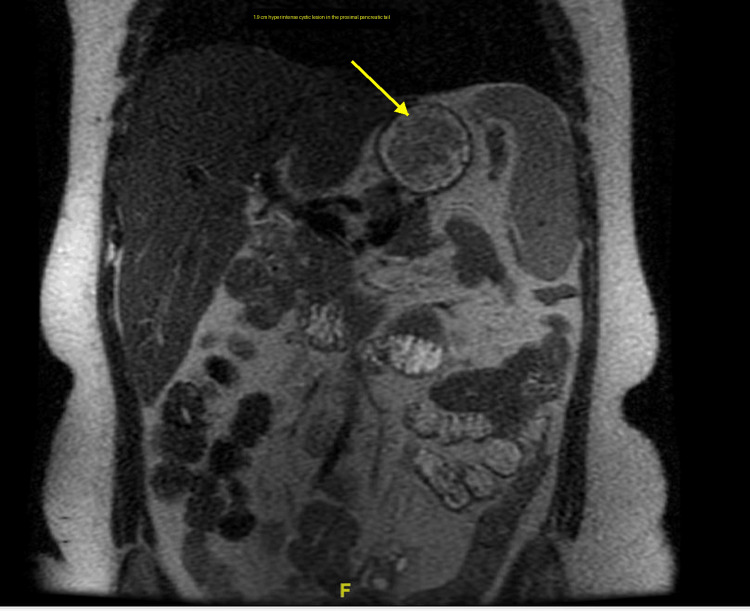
Coronal T2-weighted MRI showing a 1.9 cm hyperintense cystic lesion in the proximal pancreatic tail
